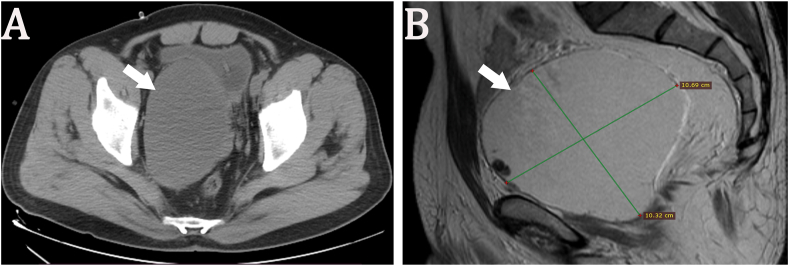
Preoperative imaging: (A) CT showed a cystic mass in the pelvis, compressing the prostate and bladder (arrow). (B) MRI showed a cystic mass measuring approximately 10 × 10 cm (arrow).
